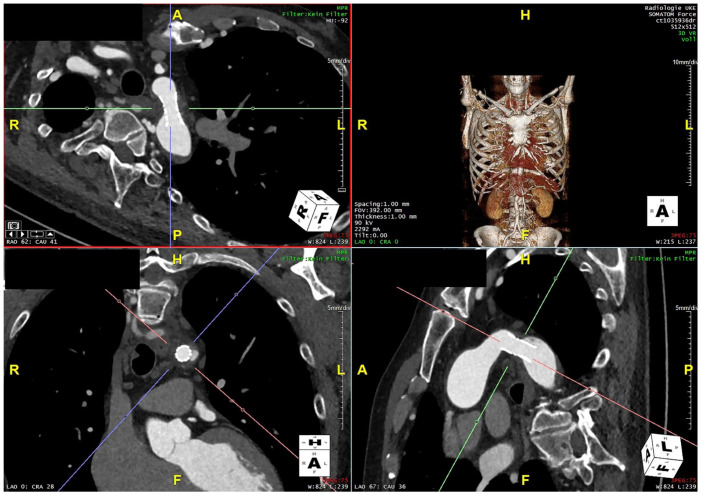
Postoperative Computed tomography angiography with a wide aortic isthmus.
